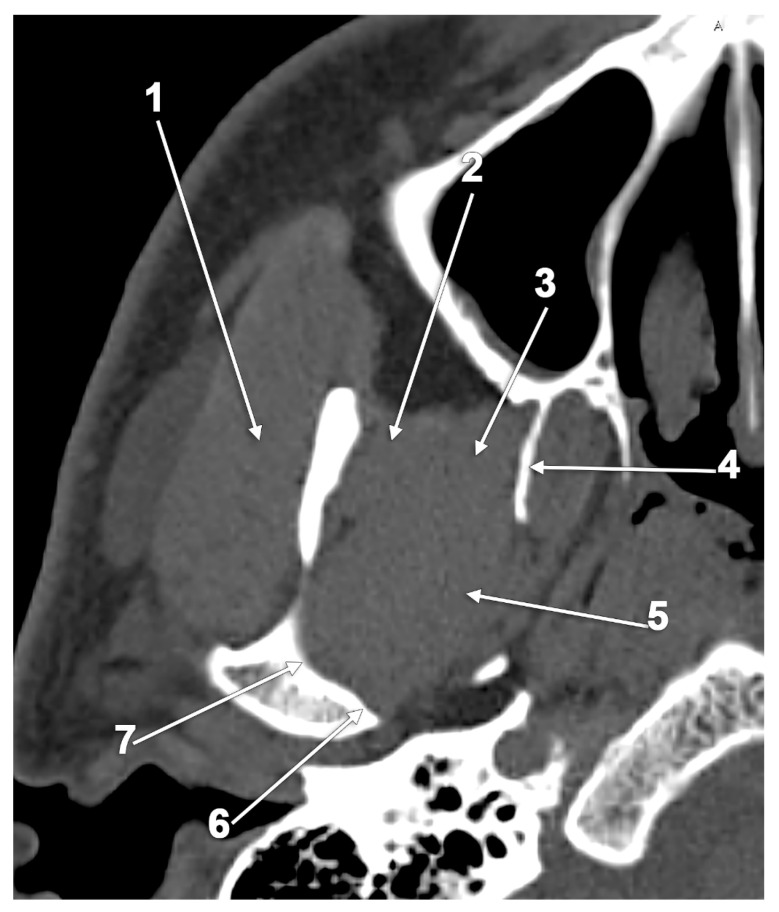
Axial CT slice through the inferior head of the lateral pterygoid muscle (ILPM): 1. masseter m.; 2. temporal m.; 3. ILPM fibers from the anterior half of the lateral pterygoid plate; 4. lateral pterygoid plate; 5. ILPM fibers from the posterior half of the lateral pterygoid plate; 6. medial condylar fovea; and 7. pterygoid fovea.
